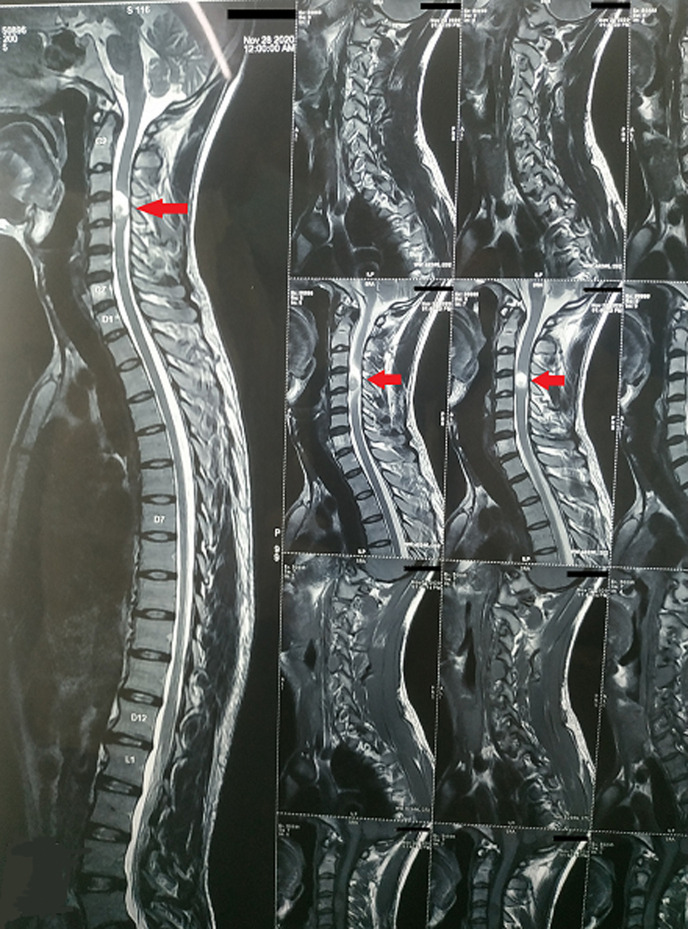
MRI findings of classical neurofibroma at the C3-C4 level
